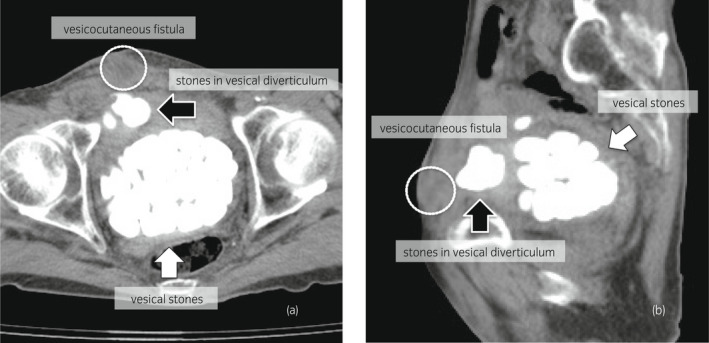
Abdominal computed tomography images (a: axial; b: sagittal) demonstrating vesical calculi (white arrows), a vesical diverticulum calculus (black arrow), and abnormal changes suspected to indicate a vesicocutaneous fistula (white circle).
